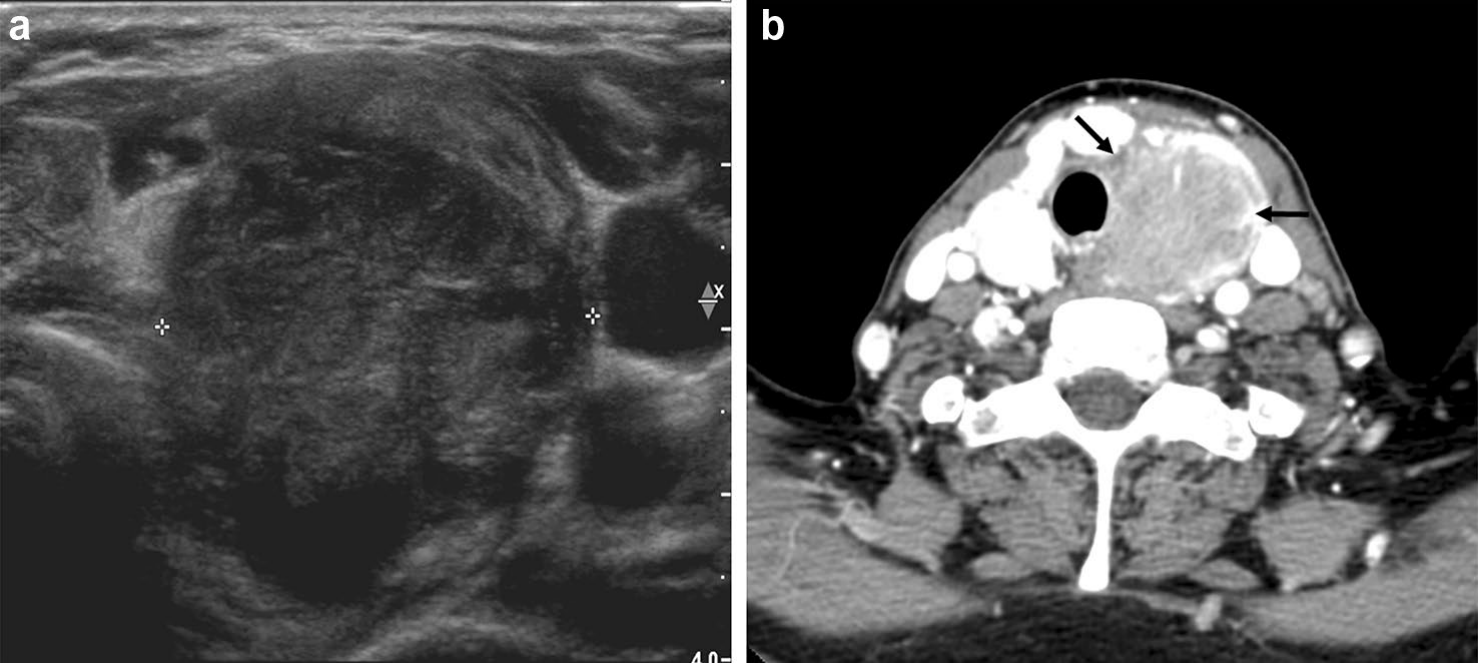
Symptomatic anaplastic thyroid carcinoma in a 58-year-old woman with swallowing difficulty. A transverse (a) sonogram shows a 3.4-cm irregular hypoechoic nodule (crosses) in the left thyroid gland, which was considered K-TIRADS 5. CT (b) reveals a heterogeneous enhanced mass (arrows) in the left thyroid gland involved in the tracheoesophageal groove.
